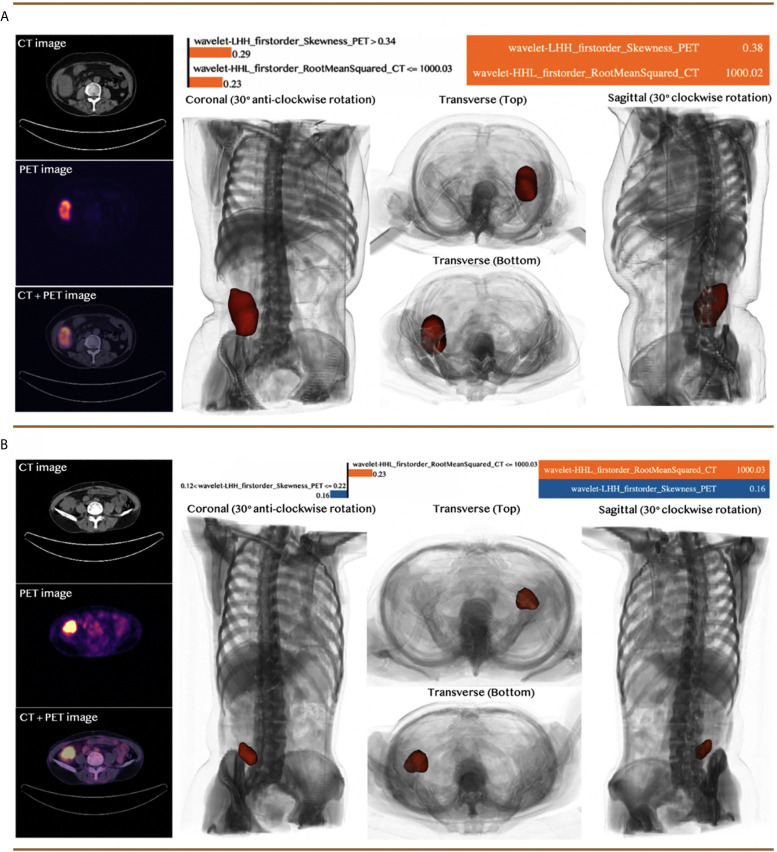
Case studies with two patients: (A) a patient with MSI-H status and (B) a patient with MSI-L or MSS status. Both patients were predicted correctly by the machine learning model. The top right section for each panel indicated the feature value of the corresponding case and the approximated feature weights interpreted by LIME. The bottom right section demonstrated the 3D model constructed based on the input CT and PET images from different viewpoints, while the red section represented the tumor of the patients.
